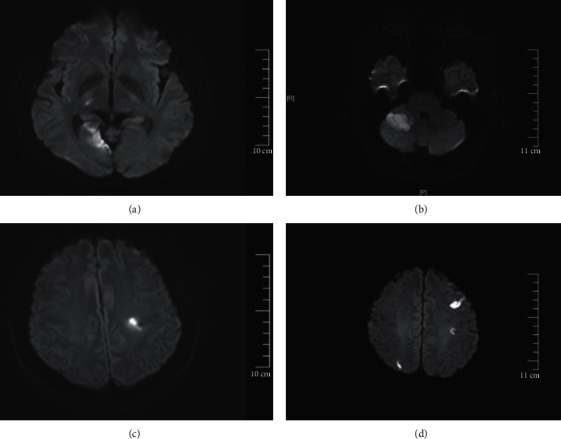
(a–d) Acute infarction in different brain regions of four patients on diffusion-weighted imaging (DWI) of MRI: right thalamus and right occipital lobe (a, patient ID: 1), right cerebellum (b, patient ID: 3), left frontoparietal junction, with a mild postinfarction hemorrhage (c, patient ID: 4), and left frontal lobe and right parietal lobe (d, patient ID: 7).
